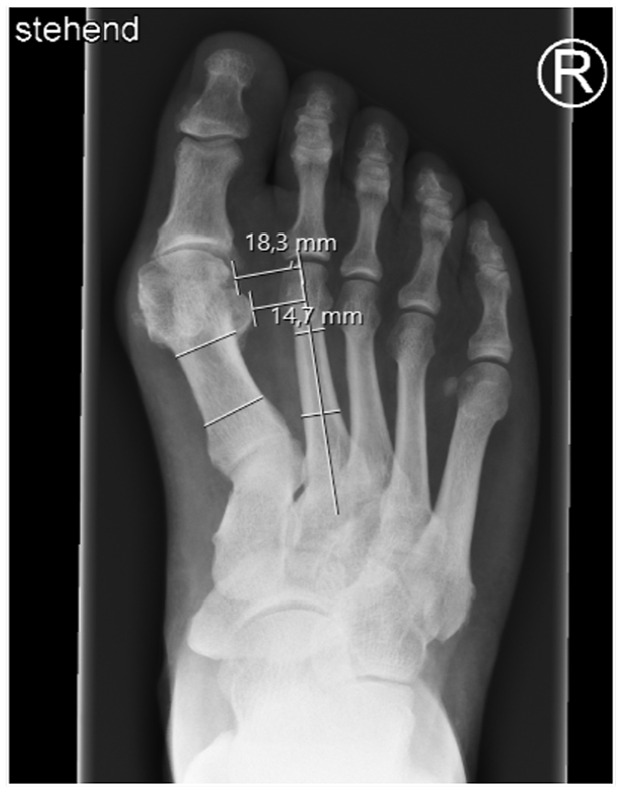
Assessment of the distance between the most lateral aspect of the head of first metatarsal and the axis of the second metatarsal (Distance MT1-MT2) and the distance of the lateral edge of the lateral sesamoid to the axis of the second metatarsal (tibial sesamoid position [TSP]) on a dorsoplantar radiograph in a standing position with full weight-bearing.
